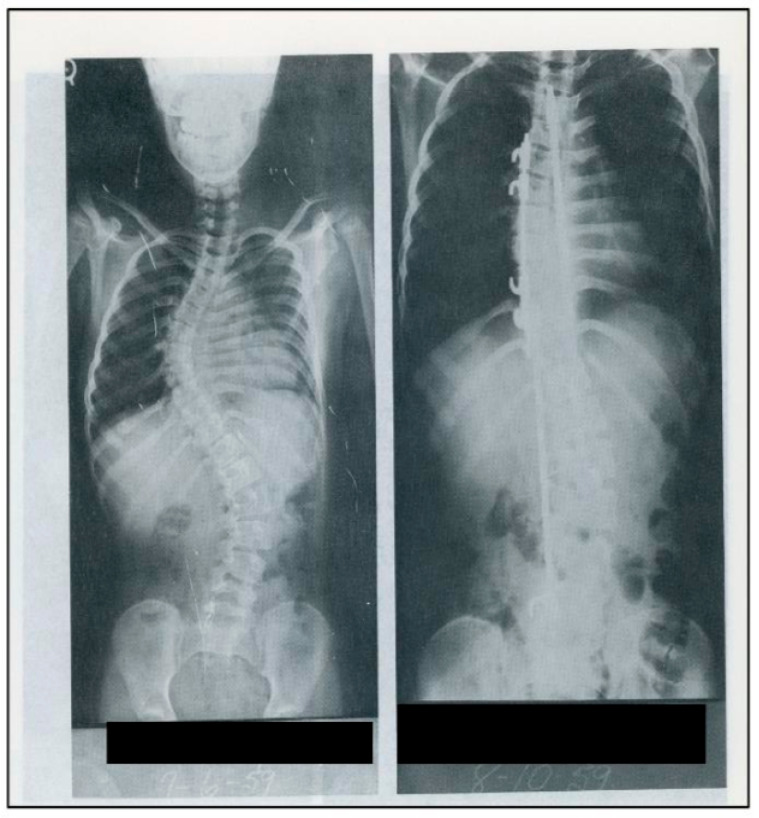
Pre- and post-operative radiographs of a 12-year-old scoliotic patient of Harrington’s after internal fixation with Harrington instrumentation, 1959 (Used with permission from the Harrington Archives, Department of History and Philosophy of Medicine, University of Kansas Medical Center).
